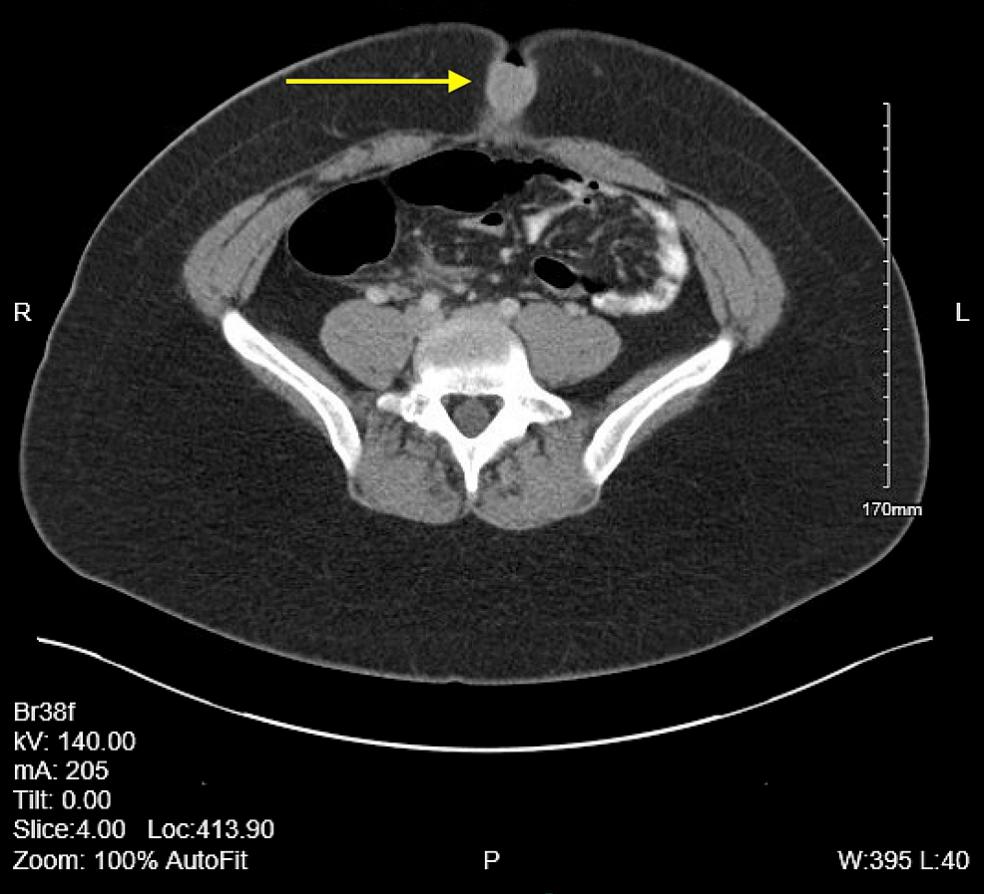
Computerized tomography (CT) scan 2
